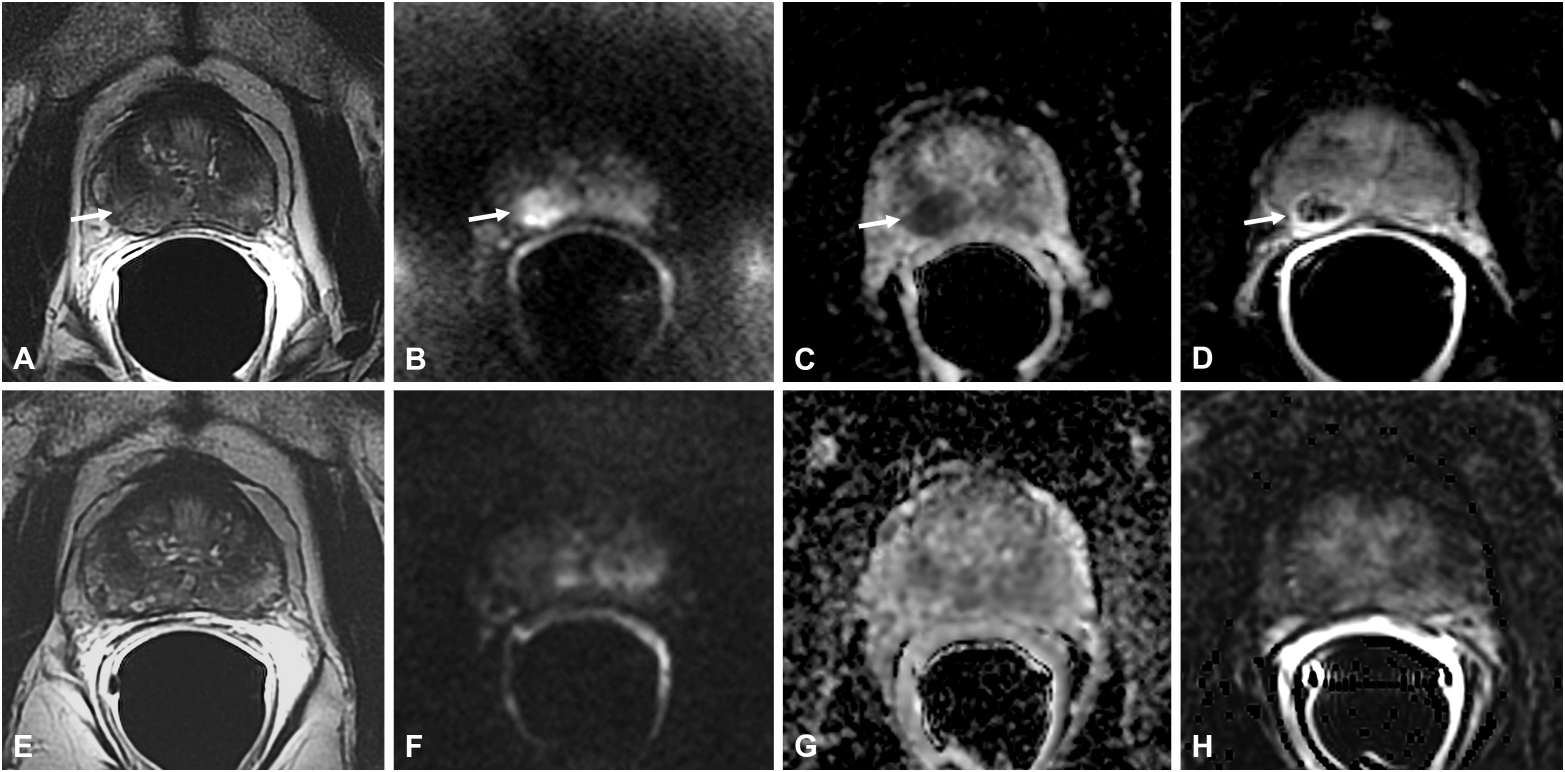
Bacillus Calmette-Guérin-induced granulomatous prostatitis in a 53-year-old patient with a PSA value of 5 ng/ml. The first mpMRI at diagnosis showed diffuse alteration of the peripheral zone (PZ) with an inhomogeneous hypointense nodule in the right middle lobe in the T2-weighted sequence (arrow in A) with hyperintensity on the DWI image (arrow in B), marked hypointensity on the ADC map (arrow in C), and typical “ring enhancement” on the DCE image (arrow in D). The corresponding sequences of the 7-month mpMRI follow-up (E–H) showed complete resolution of the aforementioned findings.
